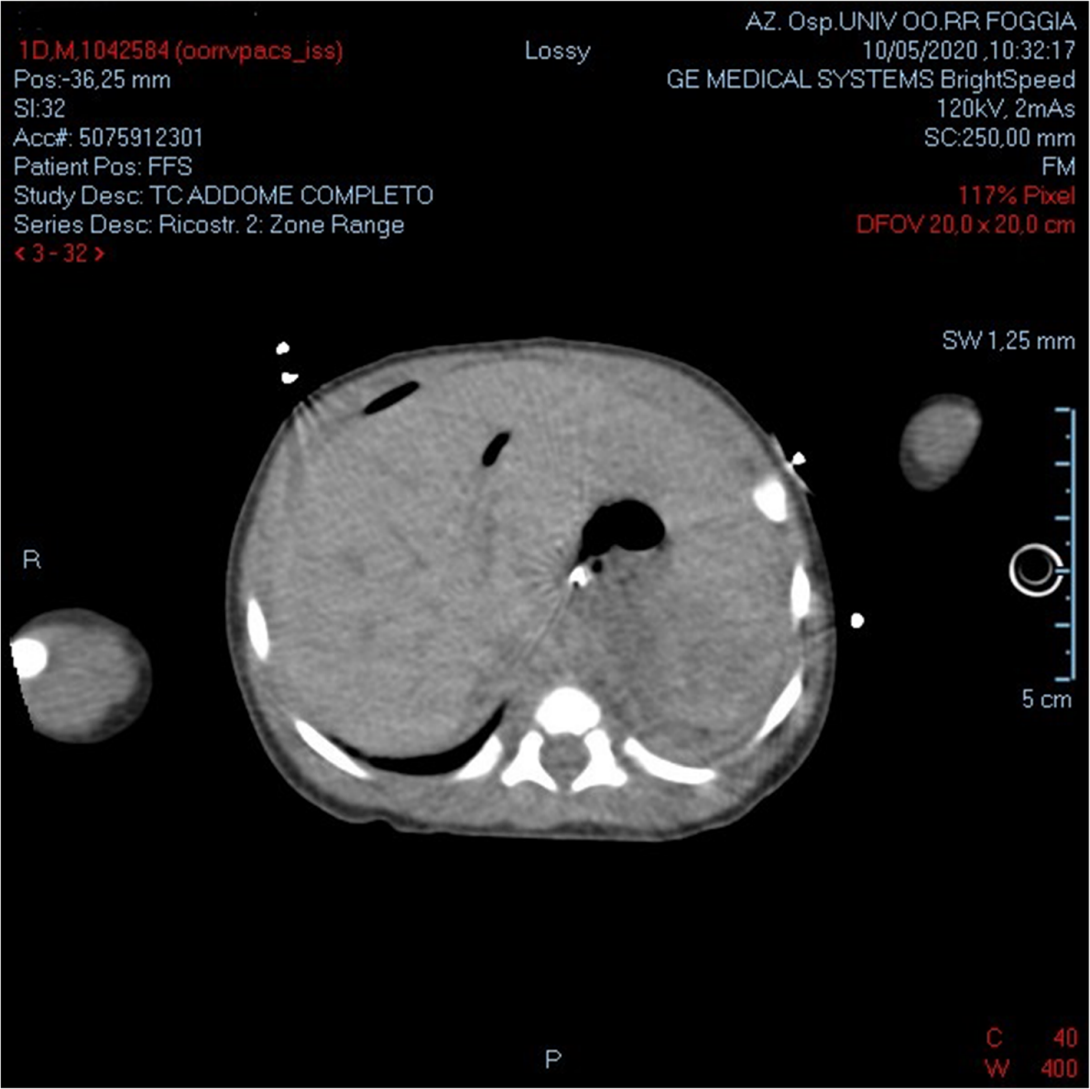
An abdominal computed tomography (CT) with contrast shows some bloody collection in the subphrenic space and between the splenic and renal veins
